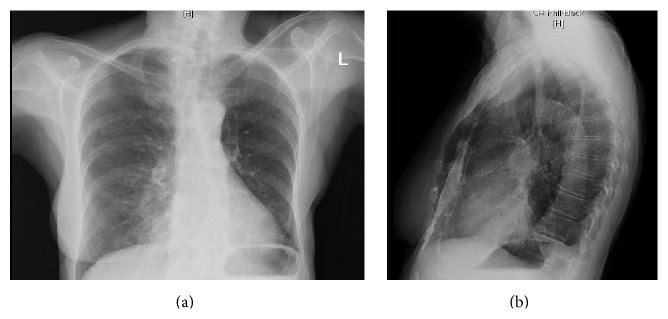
Chest X-ray findings in Case 4. Chest X-ray shows prior left lower lobectomy, stable bilateral nodules, and new consolidation versus atelectasis of the lingula.
